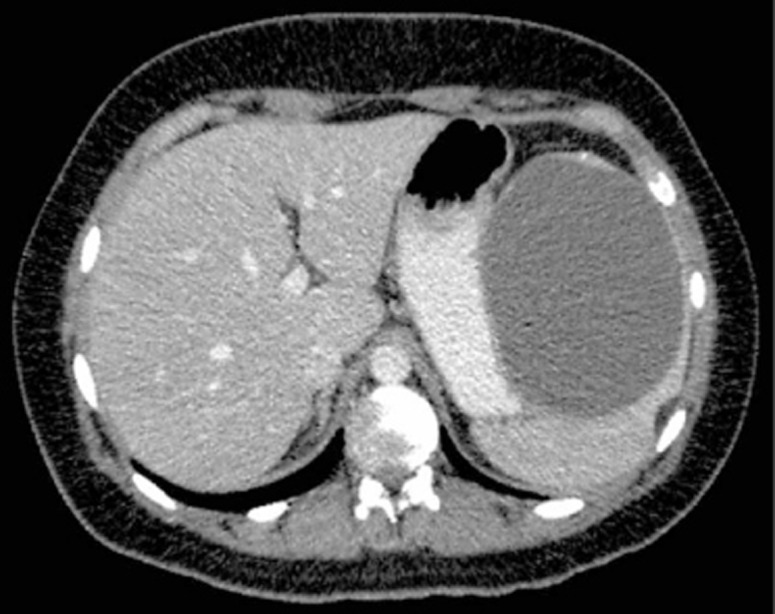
Computed tomography scan showed a cyst (11 × 8.5 cm) in the upper part of the spleen.
